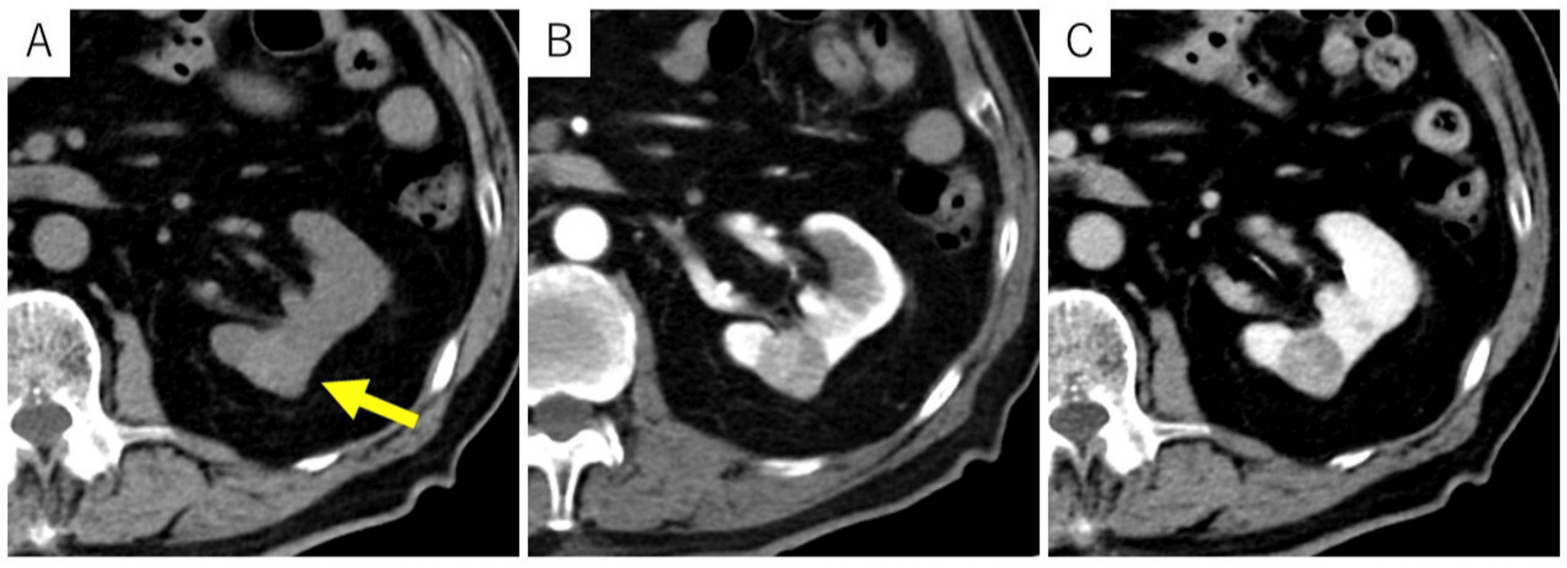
Dynamic CT of the abdomen On non-contrast CT (A), a round mass with slightly higher attenuation than the renal parenchyma was observed in the mid-portion of the left kidney. The lesion appeared predominantly solid, with internal low-attenuation areas suggesting cystic or degenerative components. The solid portion demonstrated mild enhancement in the early phase (B), followed by slight washout in the delayed phase (C) CT: computed tomography
